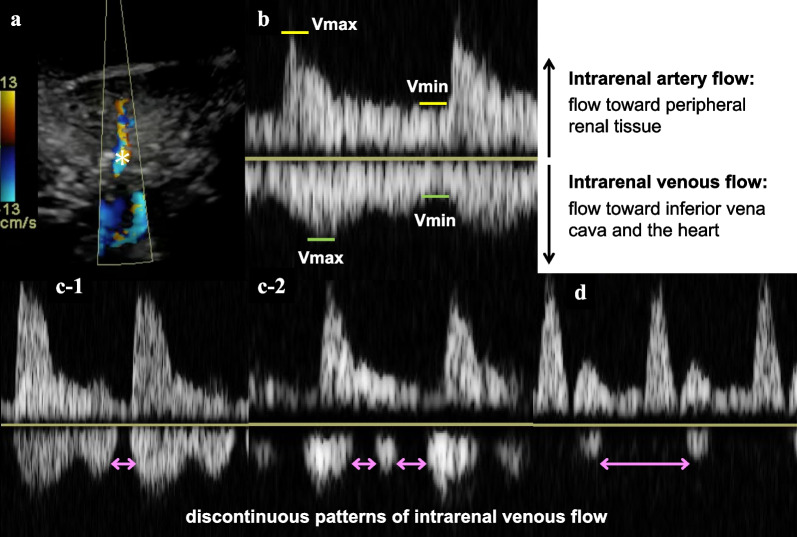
Color Doppler flow images from a right kidney. a Doppler sample volume position (*) in the inter-lobar vessels. b Intrarenal artery flow (upward Doppler signals), vein flow (downward Doppler signals) and the corresponding maximum and minimum velocity (Vmax, Vmin). The intrarenal venous flow waveforms were classified into a continuous venous flow pattern (b) or discontinuous patterns (c-1, c-2, d). Discontinuous patterns include biphasic patterns (c-1, c-2) and monophasic patterns (d) which had at least 1 phase with zero velocity during venous flow in a cardiac cycle as indicated by double-headed horizontal arrows (discontinuous time)
